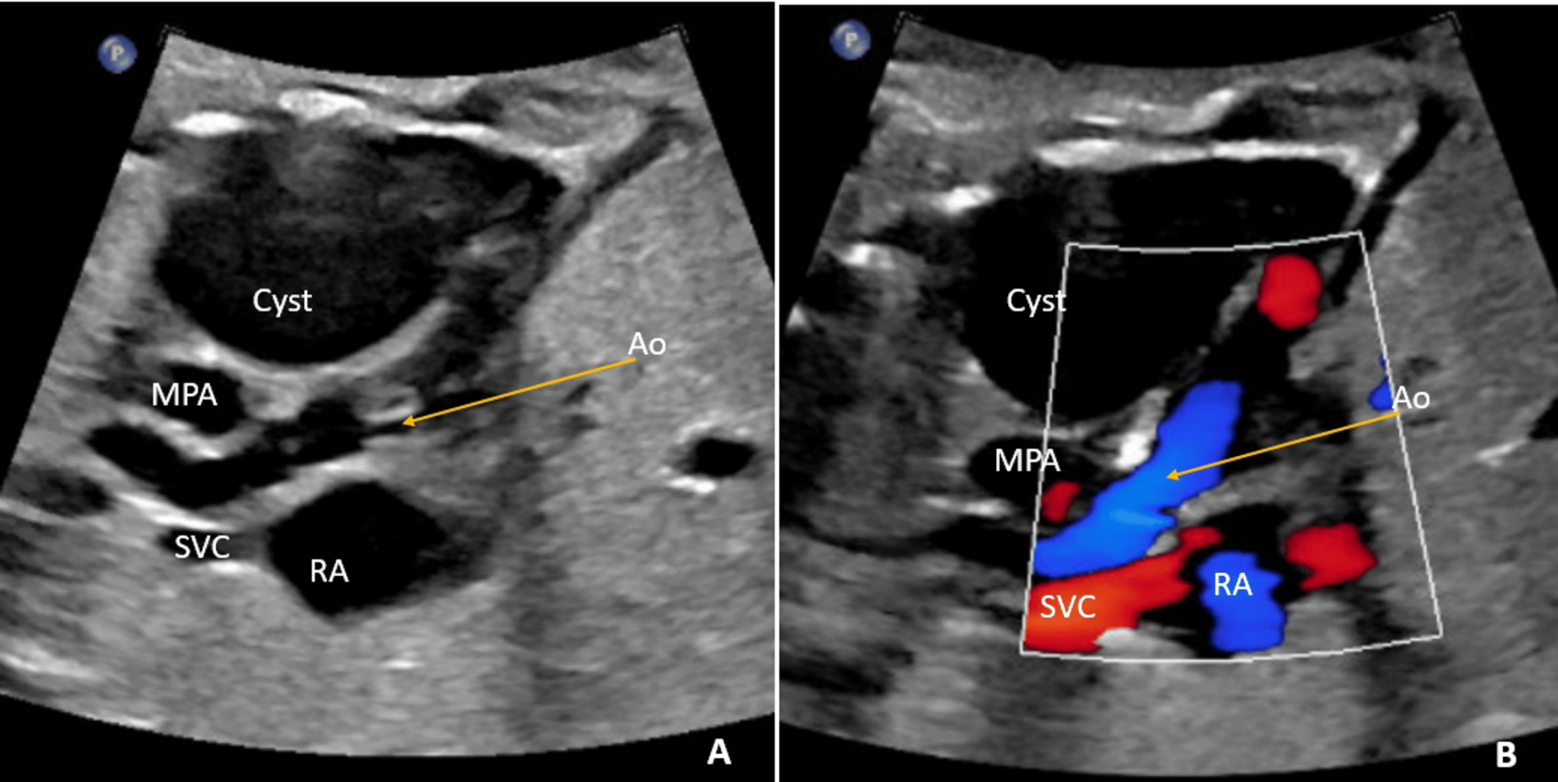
(A-B) Pre-natal ultrasound scan. A modified long-axis view of the three-vessel view showed the three vessels (SVC, aorta, and MPA) and a large single cystic mass adjacent to the left side of the fetal heart, but it failed to demonstrate communication with the LA. Ao: aortic valve; Cyst: congenital left atrial appendage aneurysm (CLAAA); MPA: main pulmonary artery; RA: right atrium; SVC: superior vena cava; LA: left atrium
